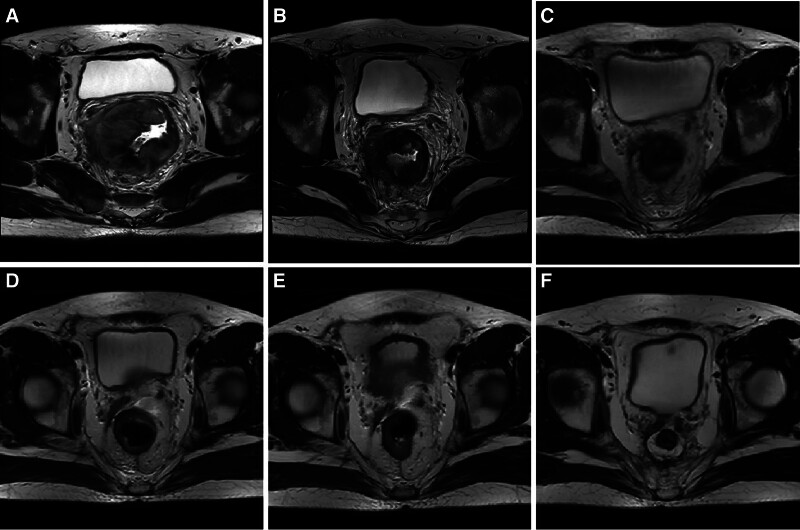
Pelvic magnetic resonance imaging (MRI) image of patients at different stages of treatment. (A) The baseline pelvic MRI image. (B) The pelvic MRI image after short-course radiotherapy and 1 cycle of chemotherapy combined with immune checkpoint inhibitors (ICIs). (C) The pelvic MRI image after 2 cycles of chemotherapy combined with ICIs. (D) The pelvic MRI image after 4 cycles of chemotherapy combined with ICIs. (E) The pelvic MRI image after 6 cycles of chemotherapy combined with ICIs. (F) The pelvic MRI image after surgery.
